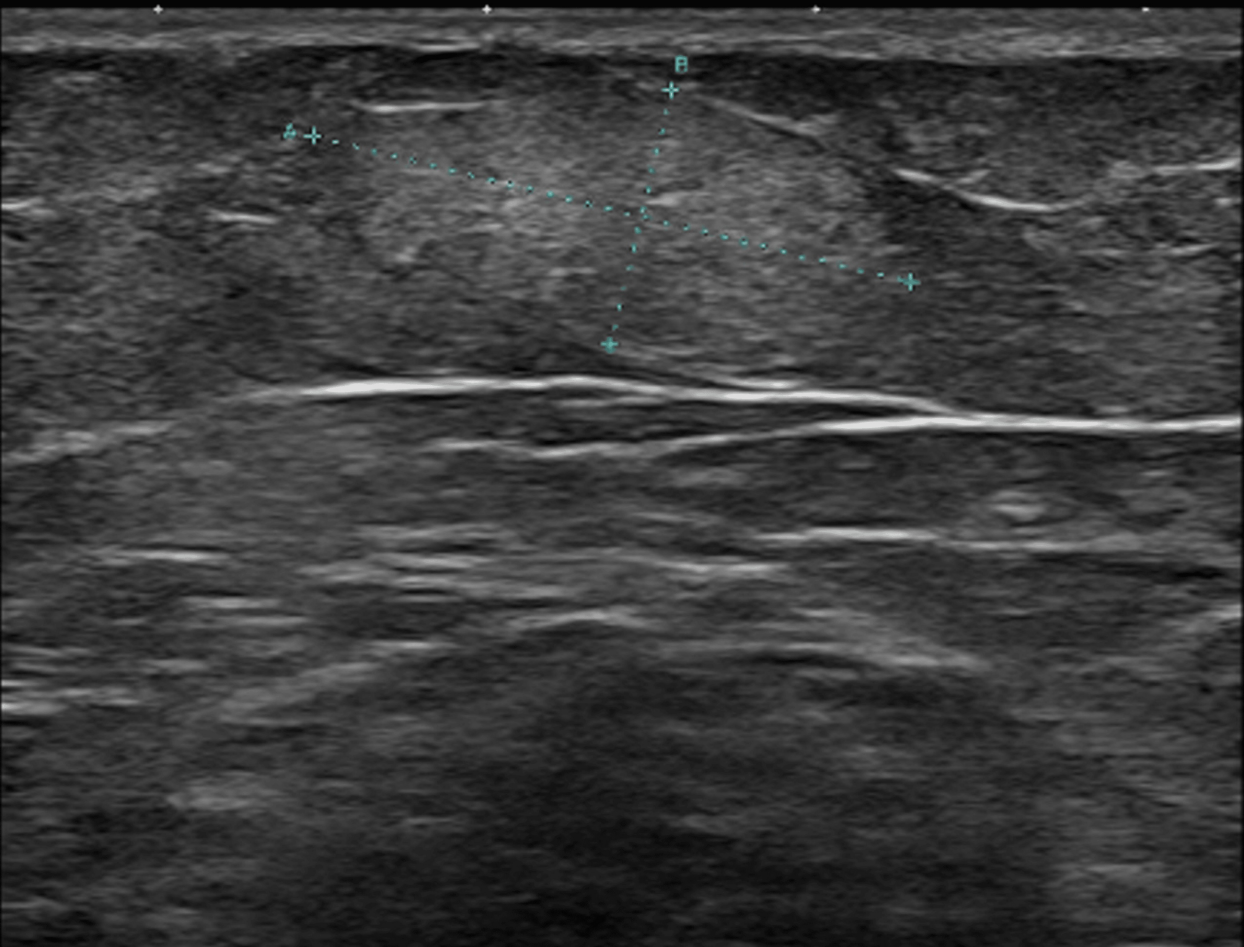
Ultrasound image of a subcutaneous lipoma measuring 1.4 × 2.0 cm. The lesion is well-circumscribed and hypoechoic, with internal echogenic strands, consistent with the typical sonographic appearance of a lipoma.
